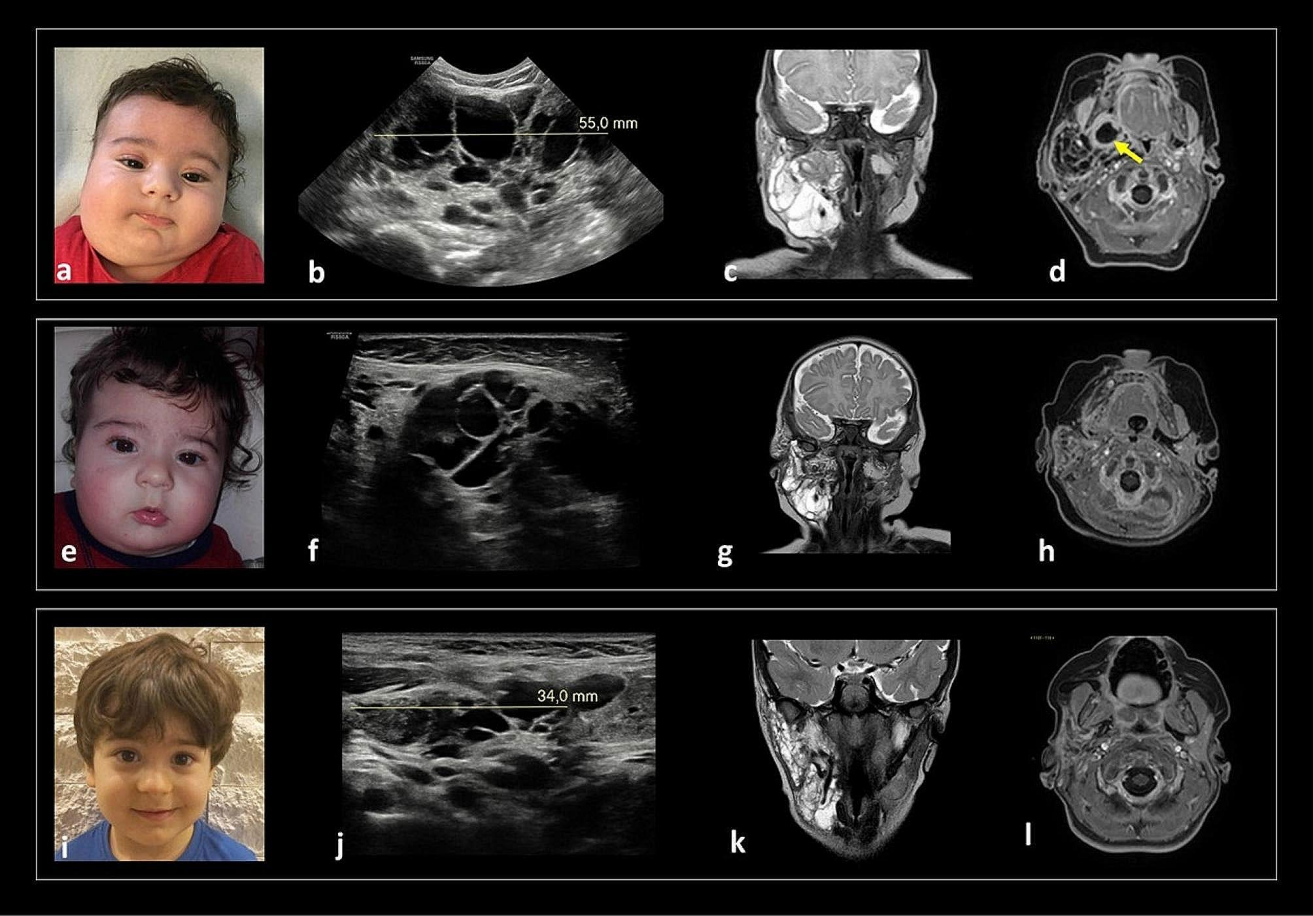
Clinical examination at 40 DOL (a), showing a lateral cervical mass on the right side of the face and neck, overlain by hyperaemic and warm skin. US (b) and MRI (c, d coronal T2w and axial T1-w after contrast medium) confirmed a large, combined LM in the right upper neck with extensive multicompartmental involvement, infiltration of the parotid, masticatory, and parapharyngeal and retropharyngeal spaces; a parapharyngeal abscess (arrow, fluid collection with thick peripheral rim of contrast enhancement), compatible with the clinically suspected superinfection, was present. Seven weeks post-sclerotherapy (5 months of age) a clinical improvement of the lesion was detected (e). US (f) and MRI (g: coronal T2w, h: axial T1-w after contrast) showed slight volumetric reduction of the LMs. The clinical examination at 2 years and 5 months showed a very mild asymmetry of the right lateral neck (i). Concomitant US showed a further reduction of the multi-chambered lesion, from 5 cm to 3.4 cm (j). The 3 years follow-up MRI (k: coronal T2w, l: axial T1-w after contrast) showed residual microcystic components in the right parotid, parapharyngeal and submandibular spaces
